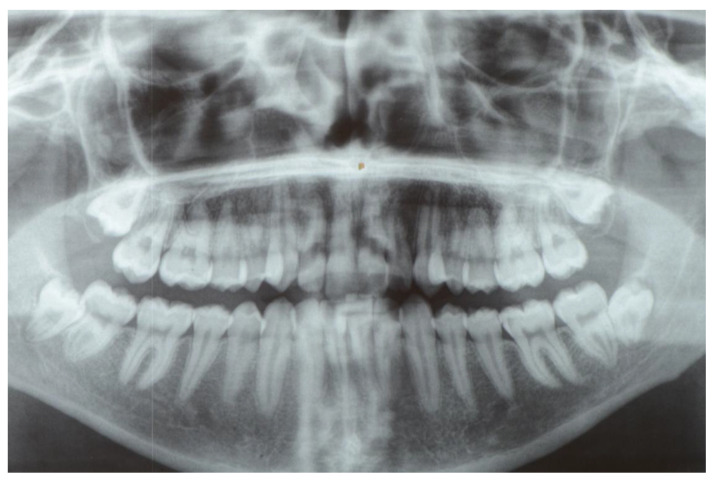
Pre-operative panoramic radiographic exam showing the impacting wisdom teeth.
